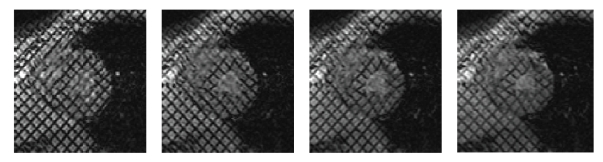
Short-Axis-Tagged MRI acquisition between end-diastole and end-systole.
